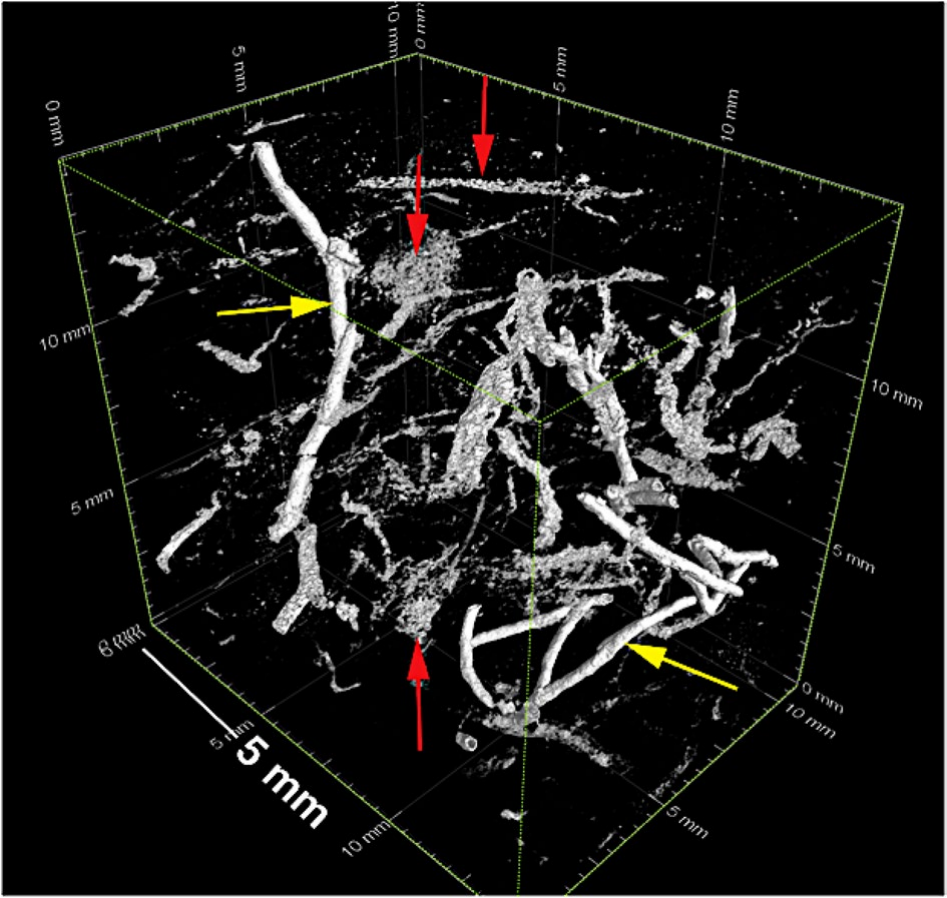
This subregion, marked with a black box in Fig. 3, has been selected for X-radiography. This higher-resolution scan reveals a three-dimensional framework of tubular pyritic structures (TPS—yellow arrows) as well as meandering, curved and zigzagging pyritic trails (PTs—red arrows) (see text for discussion).
